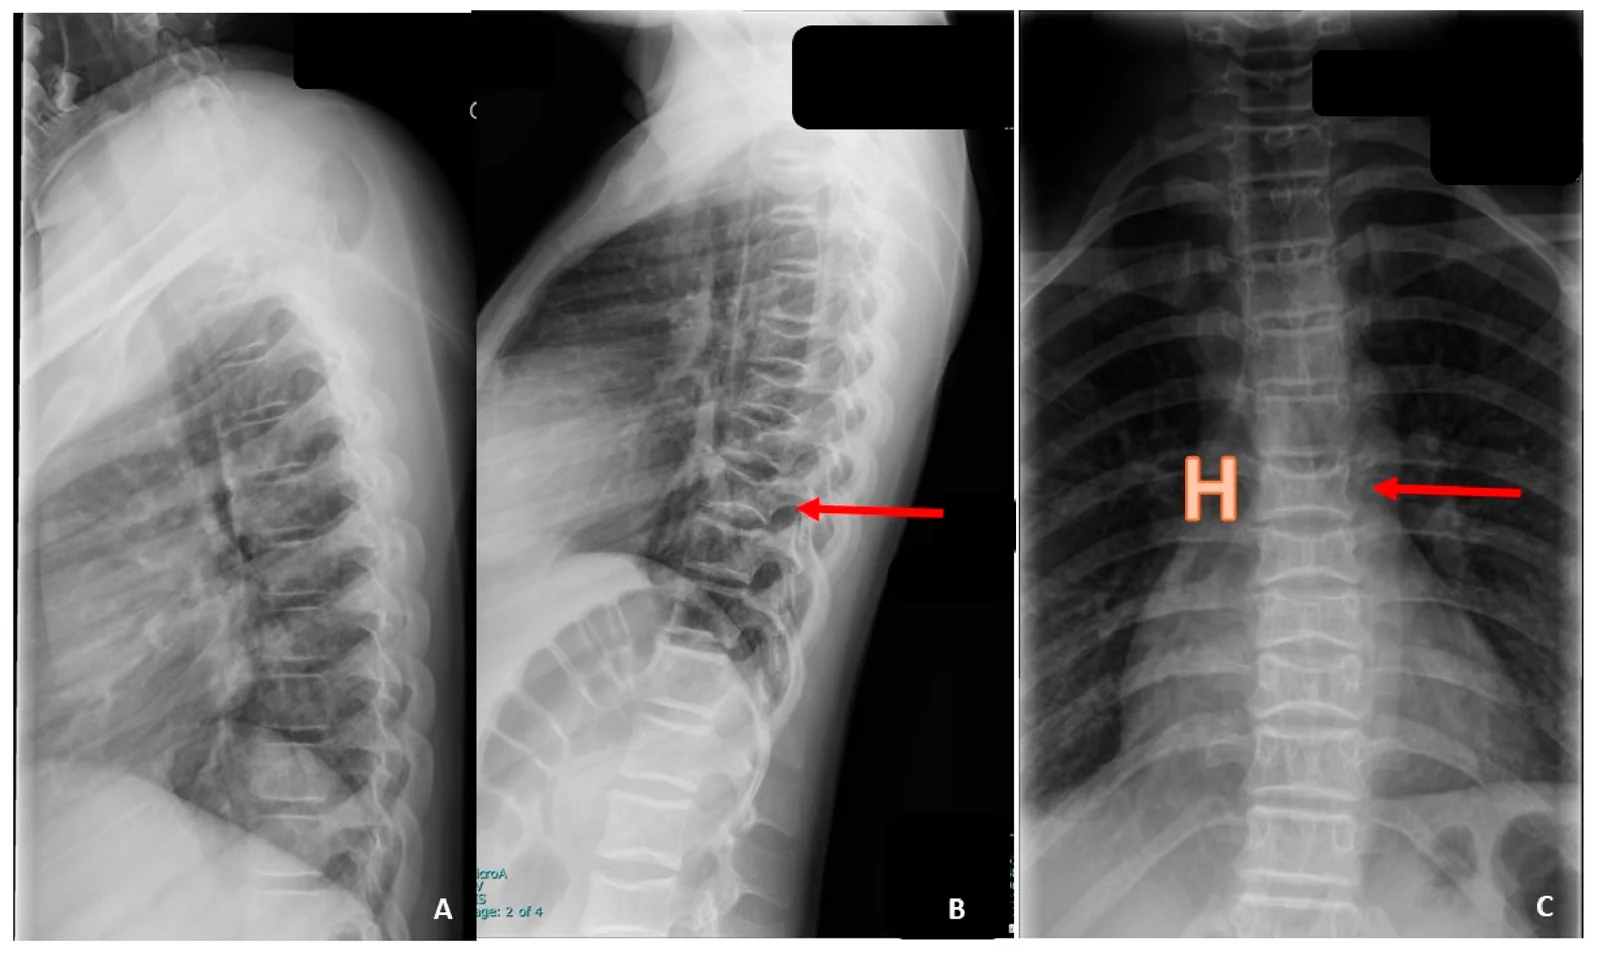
Vertebral osteonecrosis in a child with sickle cell disease. Panel (A) shows a normal lateral spine radiograph. Panels (B,C) show lateral and anteroposterior radiographs demonstrating the classic H-shaped vertebrae (red arrow) resulting from central endplate depression and osteonecrosis of the vertebral bodies in a 14-year-old child with sickle cell disease.
